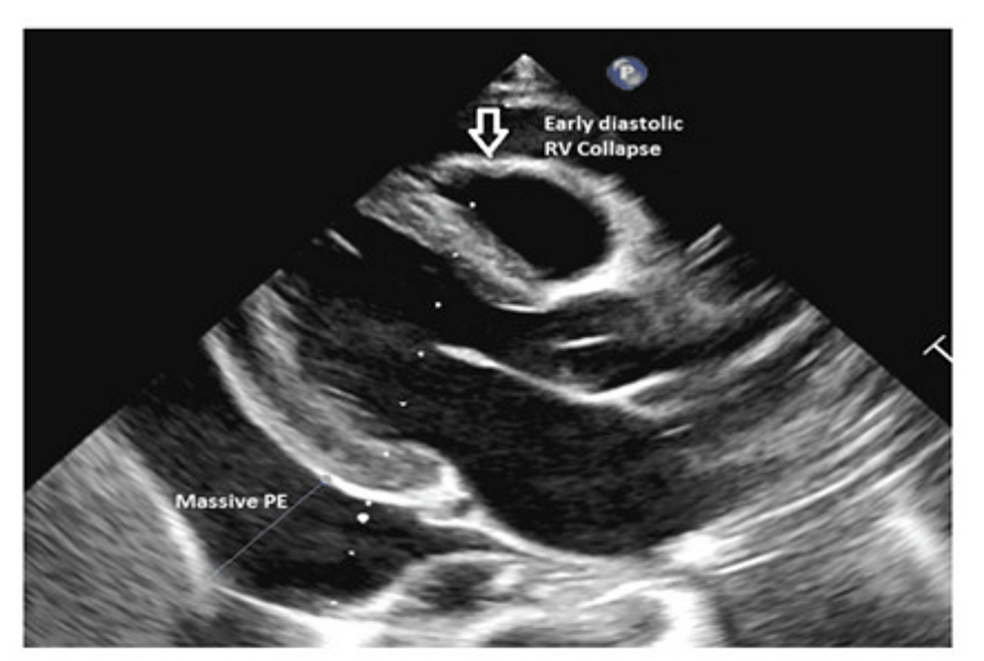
Initial echocardiography showing massive pericardial effusion and early diastolic right ventricular collapse PE: pericardial effusion; RV: right ventricular.
